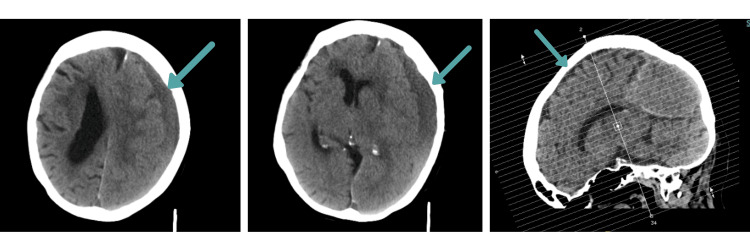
Initial CT head showing acute-on-chronic left subdural haematoma Non-contrast axial CT demonstrating left cerebral convexity subdural haematoma measuring 16 mm with posterior hyperdense layering, 9 mm midline shift, subfalcine and uncal herniation, and secondary hydrocephalus.
